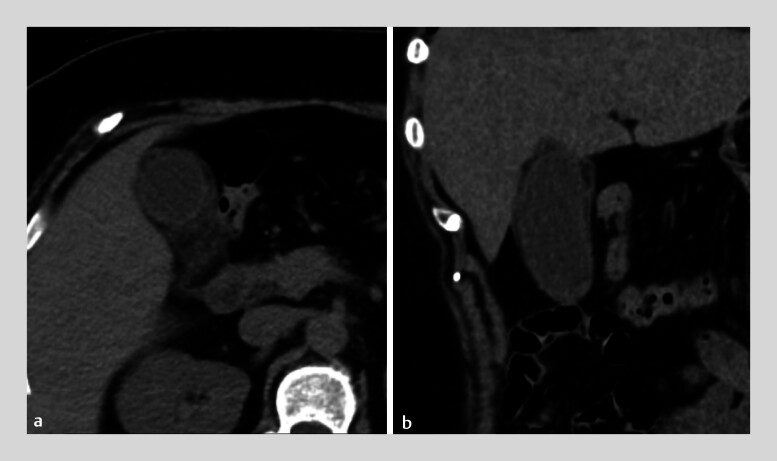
Abdominal computed tomography showing a prominent enlarged gallbladder with edematous wall thickening.
